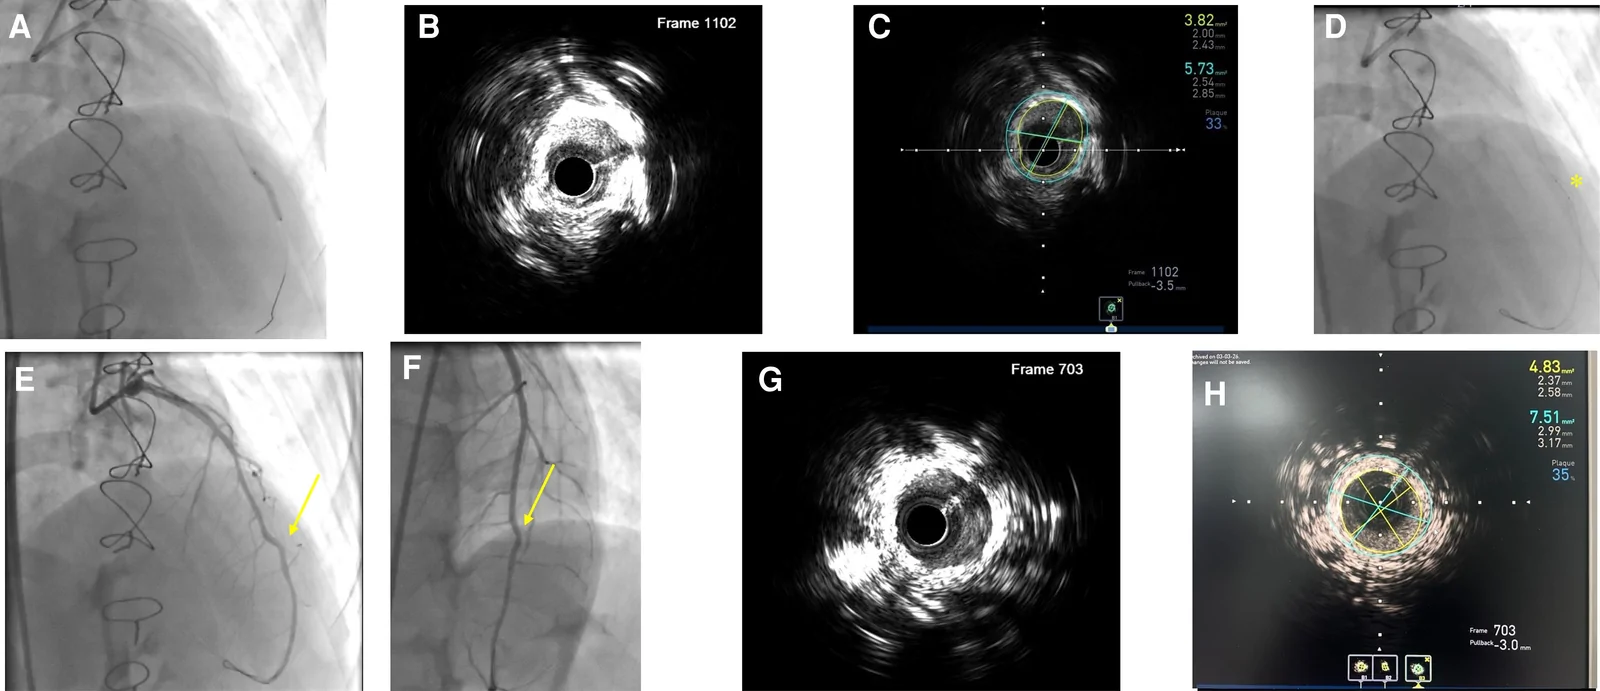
Post-percutaneous coronary intervention coronary angiography and intravascular ultrasound assessment of the left anterior descending artery lesion. *(A)* Deployment of a Xience Pro™ drug-eluting stent (2.50 × 18 mm, Abbott, USA) in the mid-left anterior descending artery. *(B)* Intravascular ultrasound was used to verify stent position and expansion after deployment. *(C)* Intravascular ultrasound shows residual stent underexpansion; the minimum stent area was estimated at 3.82 mm^2^ (reference area 5.73 mm^2^; plaque burden 33%). *(D)* A 2.5×8 mm non-compliant Solaris balloon (asterisk) (Medtronic, MN, USA) was used at high pressure (16 atmospheres). *(E, F)* Right anterior oblique cranial and left anterior oblique cranial views of the final coronary angiogram show a favourable angiographic result (arrow). *(G)* Repeat intravascular ultrasound shows full lesion coverage by the drug-eluting stent. *(H)* Final intravascular ultrasound measurement of the minimum stent area was 4.83 mm^2^ (reference area 7.51 mm^2^; plaque burden 35%; ∼64% of the reference lumen area).
